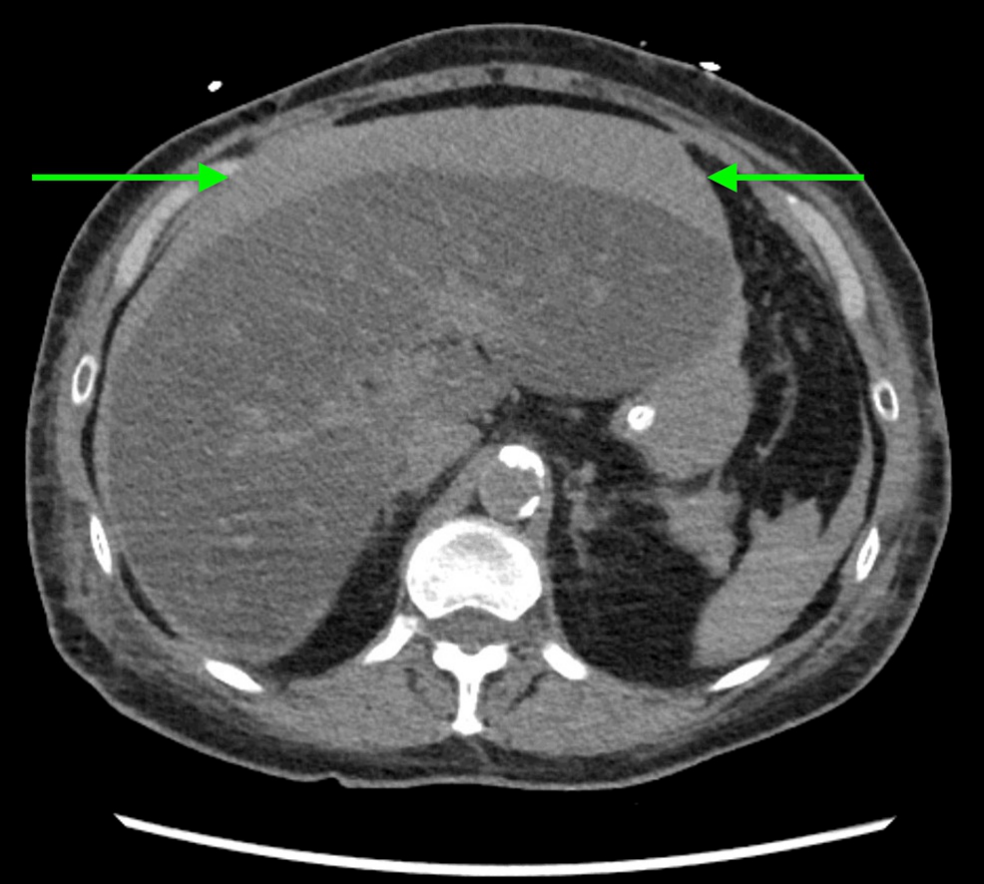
CT angiogram depicting perihepatic and capsular hemorrhage with hemoperitoneum layering in the bilateral paracolic gutters and pelvis. The perihepatic/capsular hemorrhage, as indicated by the green arrow, nearly encircles the liver, with areas up to 3 cm thick anteriorly.
